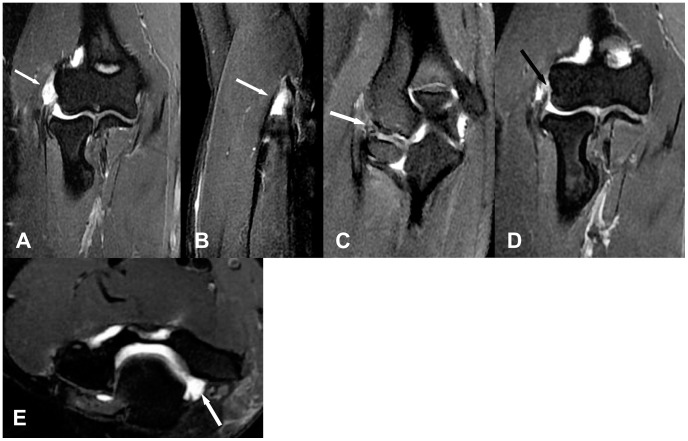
A 40-year-old female with right elbow pain about 3 months. (A,B) Coronal and Saggital fat–suppressed FSE T2-weighted image shows abnormal fluid signal intensity affecting more than 80% of the CET thickness, which suggests severe injury (white arrow). (C) Coronal fat-suppressed FSE T2-weighted image shows thinning and line-like high signal intensity across the LCUL origin, a finding suggestive of severe injury (white arrow). (D) Coronal fat-suppressed FSE T2-weithted image shows complete absence of the proximal fibers of the RCL (black arrow). demonstrating full-thickness tear of the RCL (white arrow). (E) Axial fat-suppressed FSE T2-weighted image shows joint effusion (white arrow).
